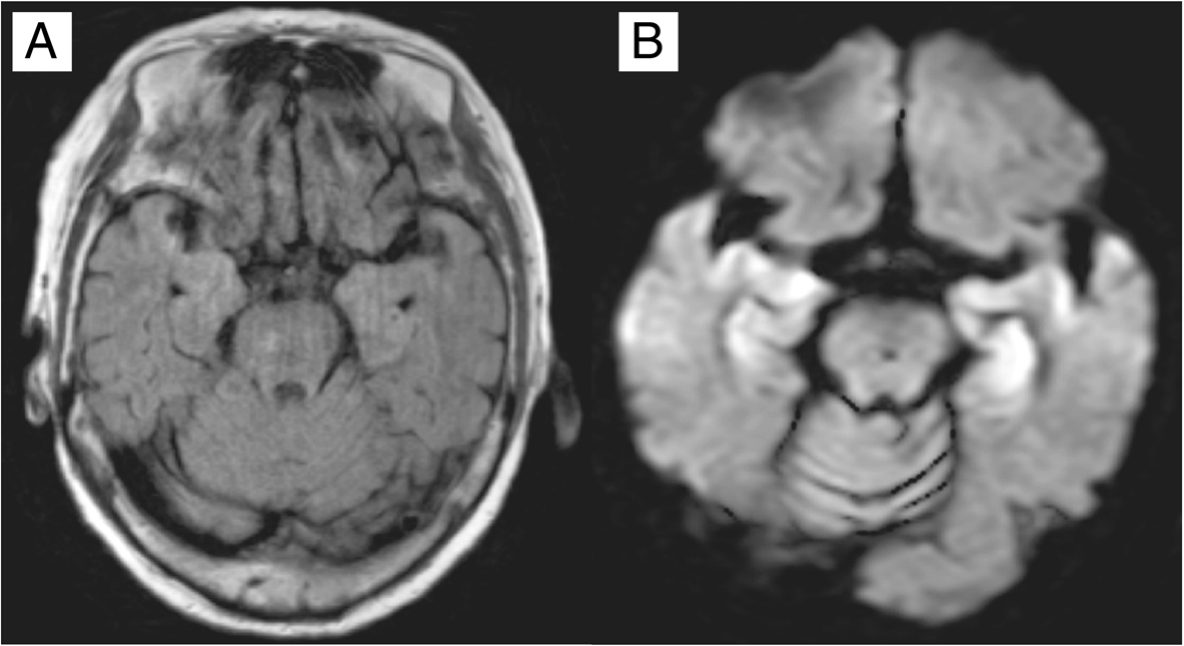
Magnetic resonance imaging of the brain upon onset of symptoms. a Coronal T2-weighted magnetic resonance imaging of the brain revealed high intensity areas bilaterally in the temporal lobes. b Diffusion-weighted imaging revealed enhanced high intensity areas corresponding to the bilateral temporal lobes
